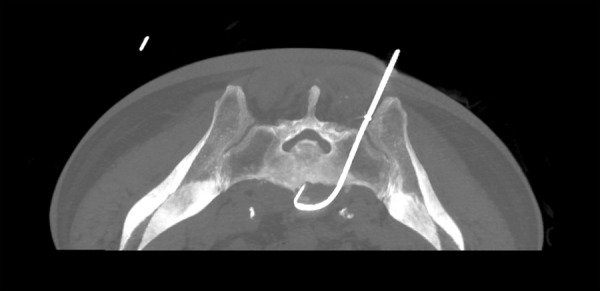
Transverse CT image showing drainage catheter placement.
